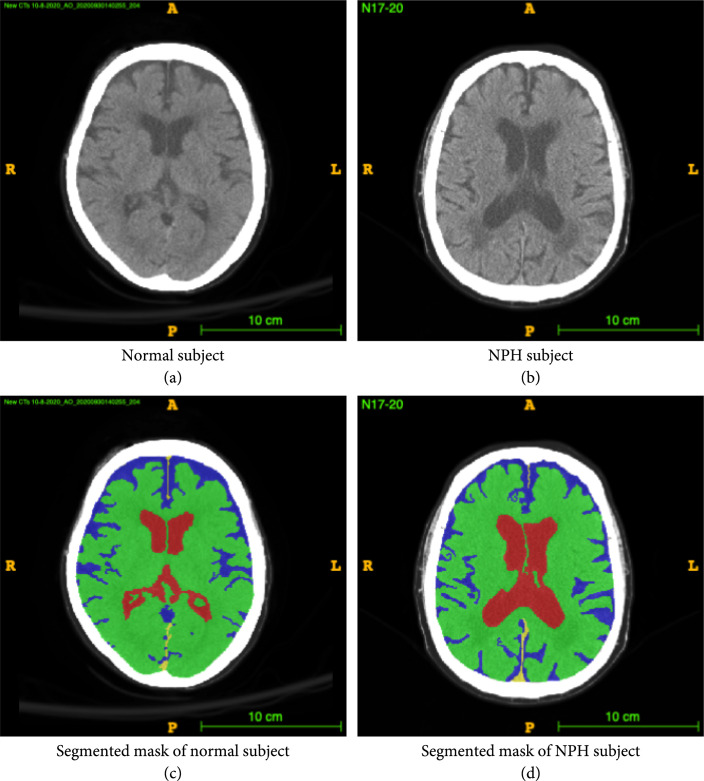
Example CT image slice of area with widest frontal horns in (a) a normal subject and (b) a subject diagnosed with NPH. (c) and (d) are masks overlaid on the original image indicating the ventricles (red), gray-white matter (green), and subarachnoid space (blue). In this example, the width of the frontal horns appear similar in the normal subject and the NPH subject, but the total volume of the ventricles differs. This is one example of a case where 3D volumetric measurements may be helpful in differentiating potential patients with NPH from those without NPH. These annotations are carefully created from the 3D data and are available to the public.
